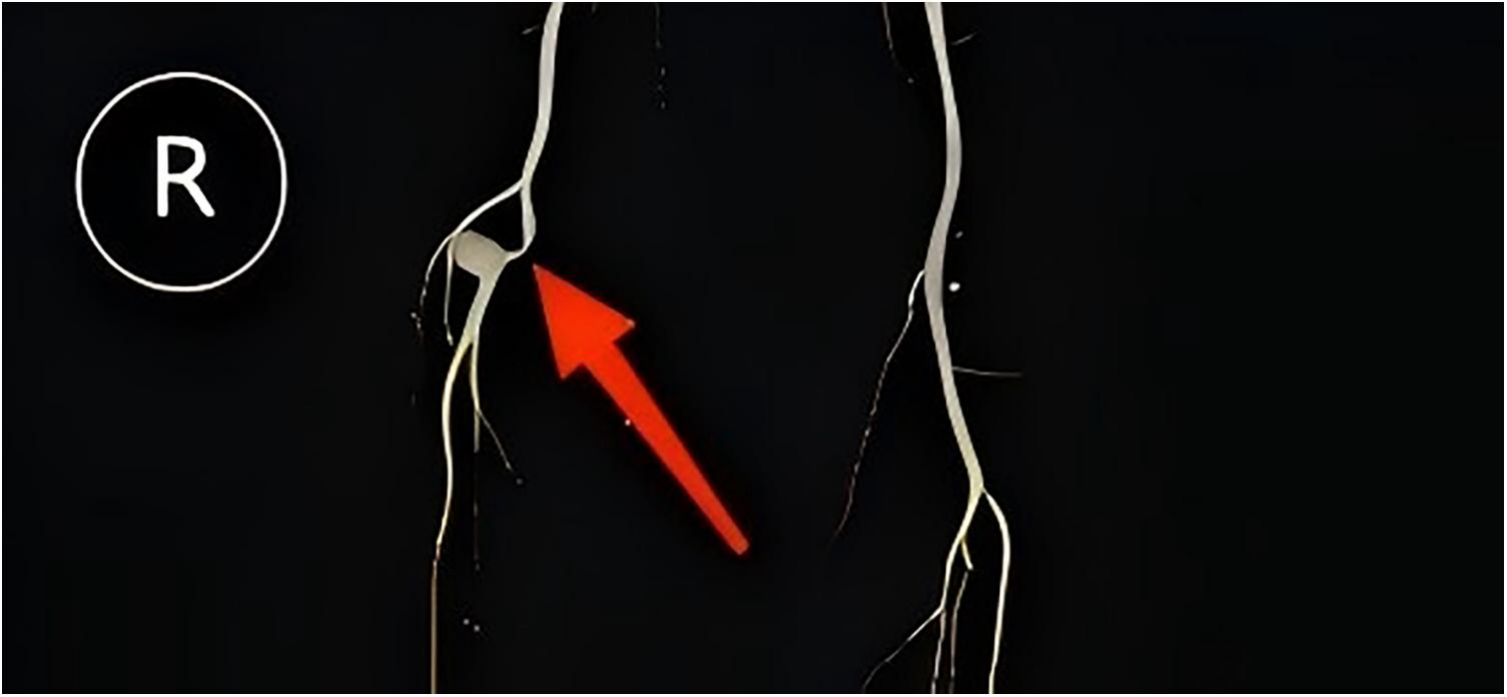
CT angiography showing the aneurysm with a maximum diameter of 3.7 cm and moderate-to-severe extrinsic stenosis of the proximal parent artery. Arrow indicates the compressed and stenotic segment above the popliteal artery aneurysm.
